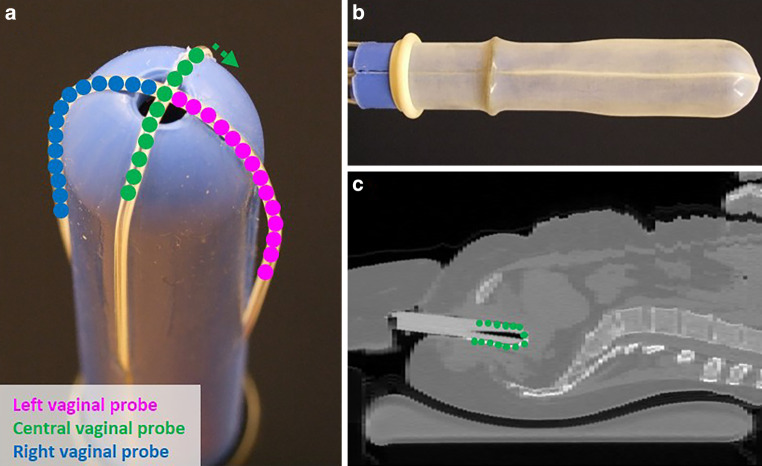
a Silicone elastomer mold applicator (diameter 25 mm) used to fixate intravaginal thermometry probes to measure target temperatures during hyperthermia treatments of cervical cancer patients. Three 14-sensor thermocouple probes (*left*, *central*, *right*) with 5‑mm sensor spacing are positioned along the grooves in the mold applicator. b The mold applicator and the thermocouple probes are covered with a condom before insertion into the patient’s vagina. c Hyperthermia planning CT scan in hyperthermia treatment position with thermometry in situ
